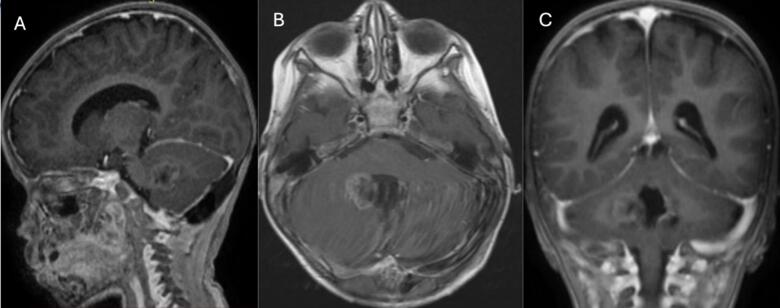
Magnetic resonance imaging of the brain: First MRI done after the first surgical intervention.
